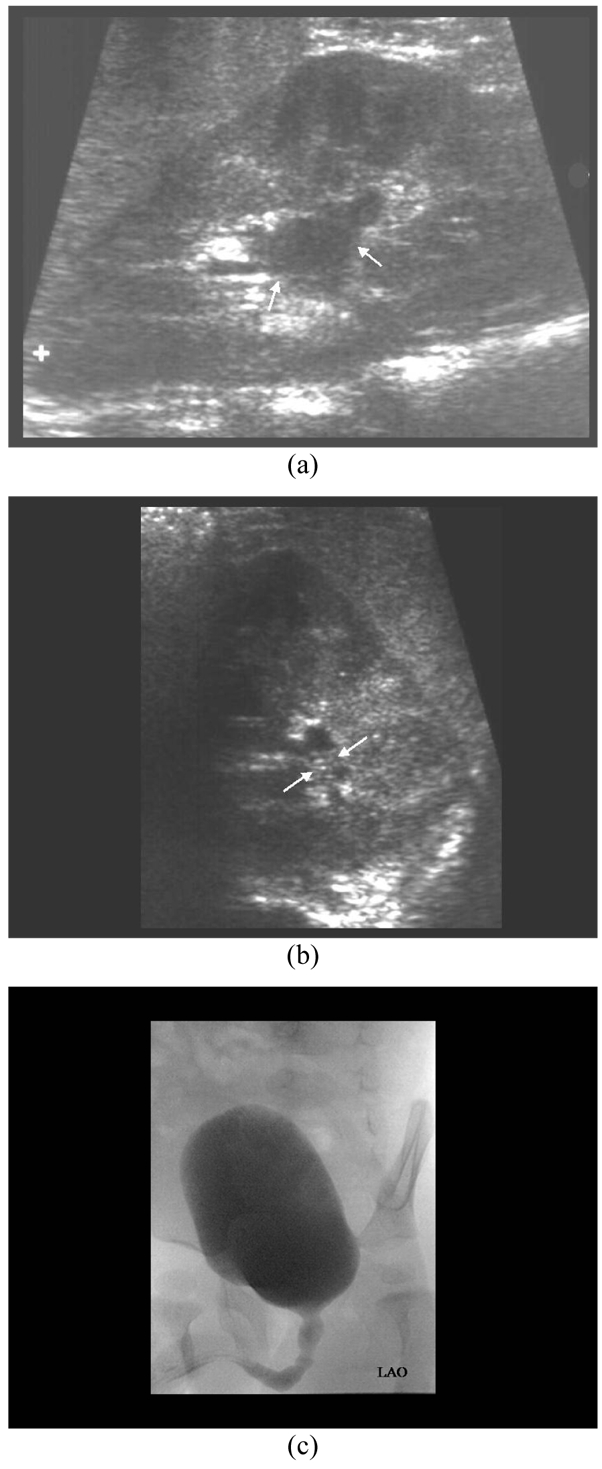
An eighteen months-old boy had history of urinary tract infection and positive urine culture for E.coli; a) Renal sonogram shows left mild hydronephrosis (arrows); b) ECS (in axial plane) demonstrates presence of microbubbles (arrows) in the dilated PCS without increase in PCS dilatation; c) MCU is normal.
